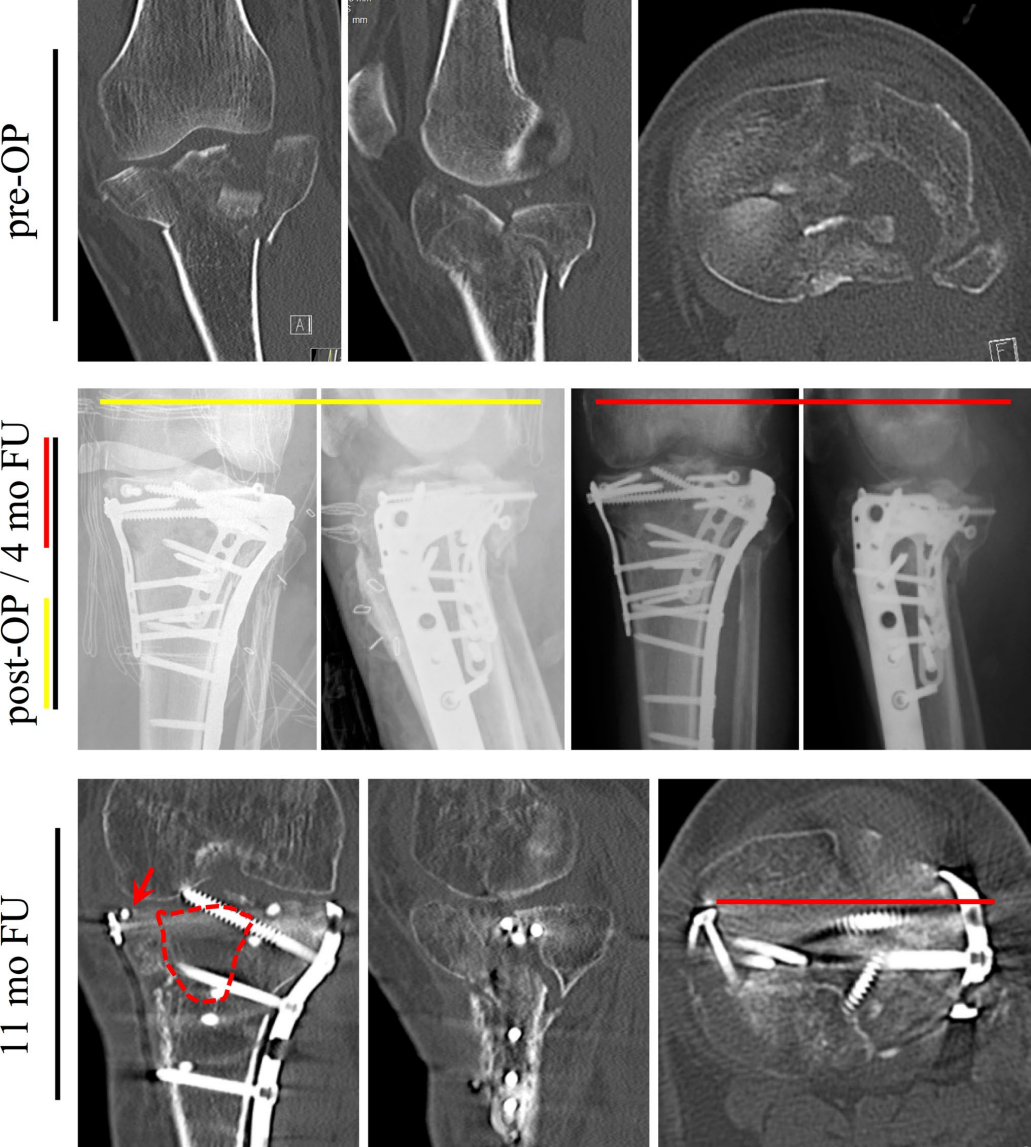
A 50-year-old male with a bicondylar TPF (AO 41-type C3, medial split, lateral comminution involving the tibial spine and the most severe depression in the postero-latero-central segment, *pre-OP*) after a fall due to alcohol intoxication. A postoperative X-ray of the lateral and posterolateral reduction with individual plate fixation in addition to anatomic reduction and medial plate fixation of the medial tibial plateau (yellow head line). Medially, an additional jail screw supported subchondral fixation (*post-OP)*. The 4-month follow-up (FU) showed secondary loss of reduction (red arrow in the anterior–posterior view) with a substantial subchondral bone defect (red dotted line), secondary intraarticular screw location, and an osteopenic bone stock (*4-mo FU*). The sagittal and axial views revealed an unfortunate screw placement into the fracture gap without providing sufficient stabilization. While the antero-posterior jail screw might not have had enough osteosynthetic subchondral bone support, there was no posteromedial buttress. Comorbidities included chronic hypocalcemia, chronic alcohol abuse, and long-term smoking
